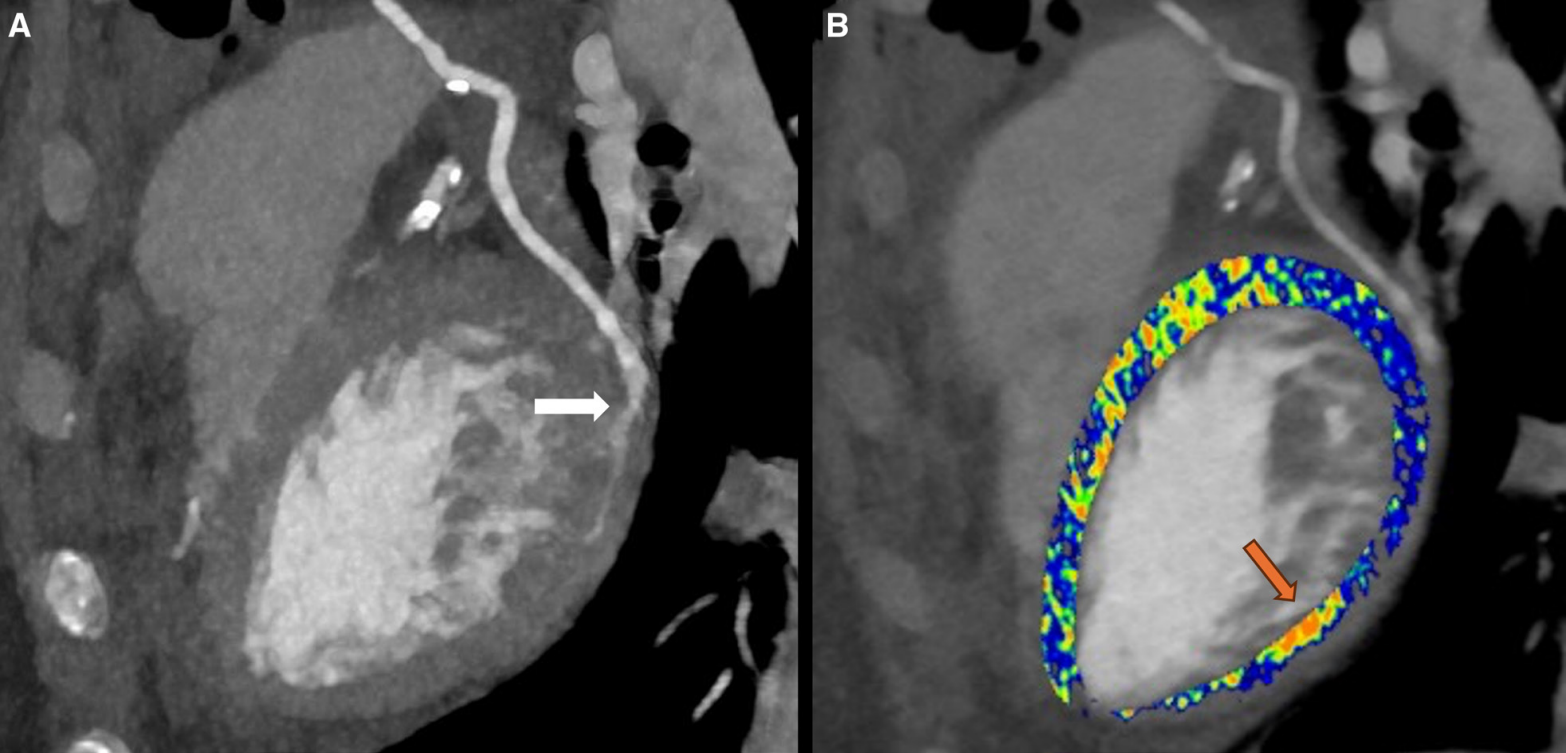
Photon-counting detector computed tomography angiography of the venous graft from the aorta to the obtuse marginal branch of the left circumflex coronary artery with corresponding extracellular volume (ECV) map. A, At the level of anastomosis (white arrow), stenosis of the venous graft is visualized and distal filling of the left circumflex coronary artery is impaired. B, A corresponding ECV map reveals focal ECV elevation (orange arrow) distal to the obstruction.
